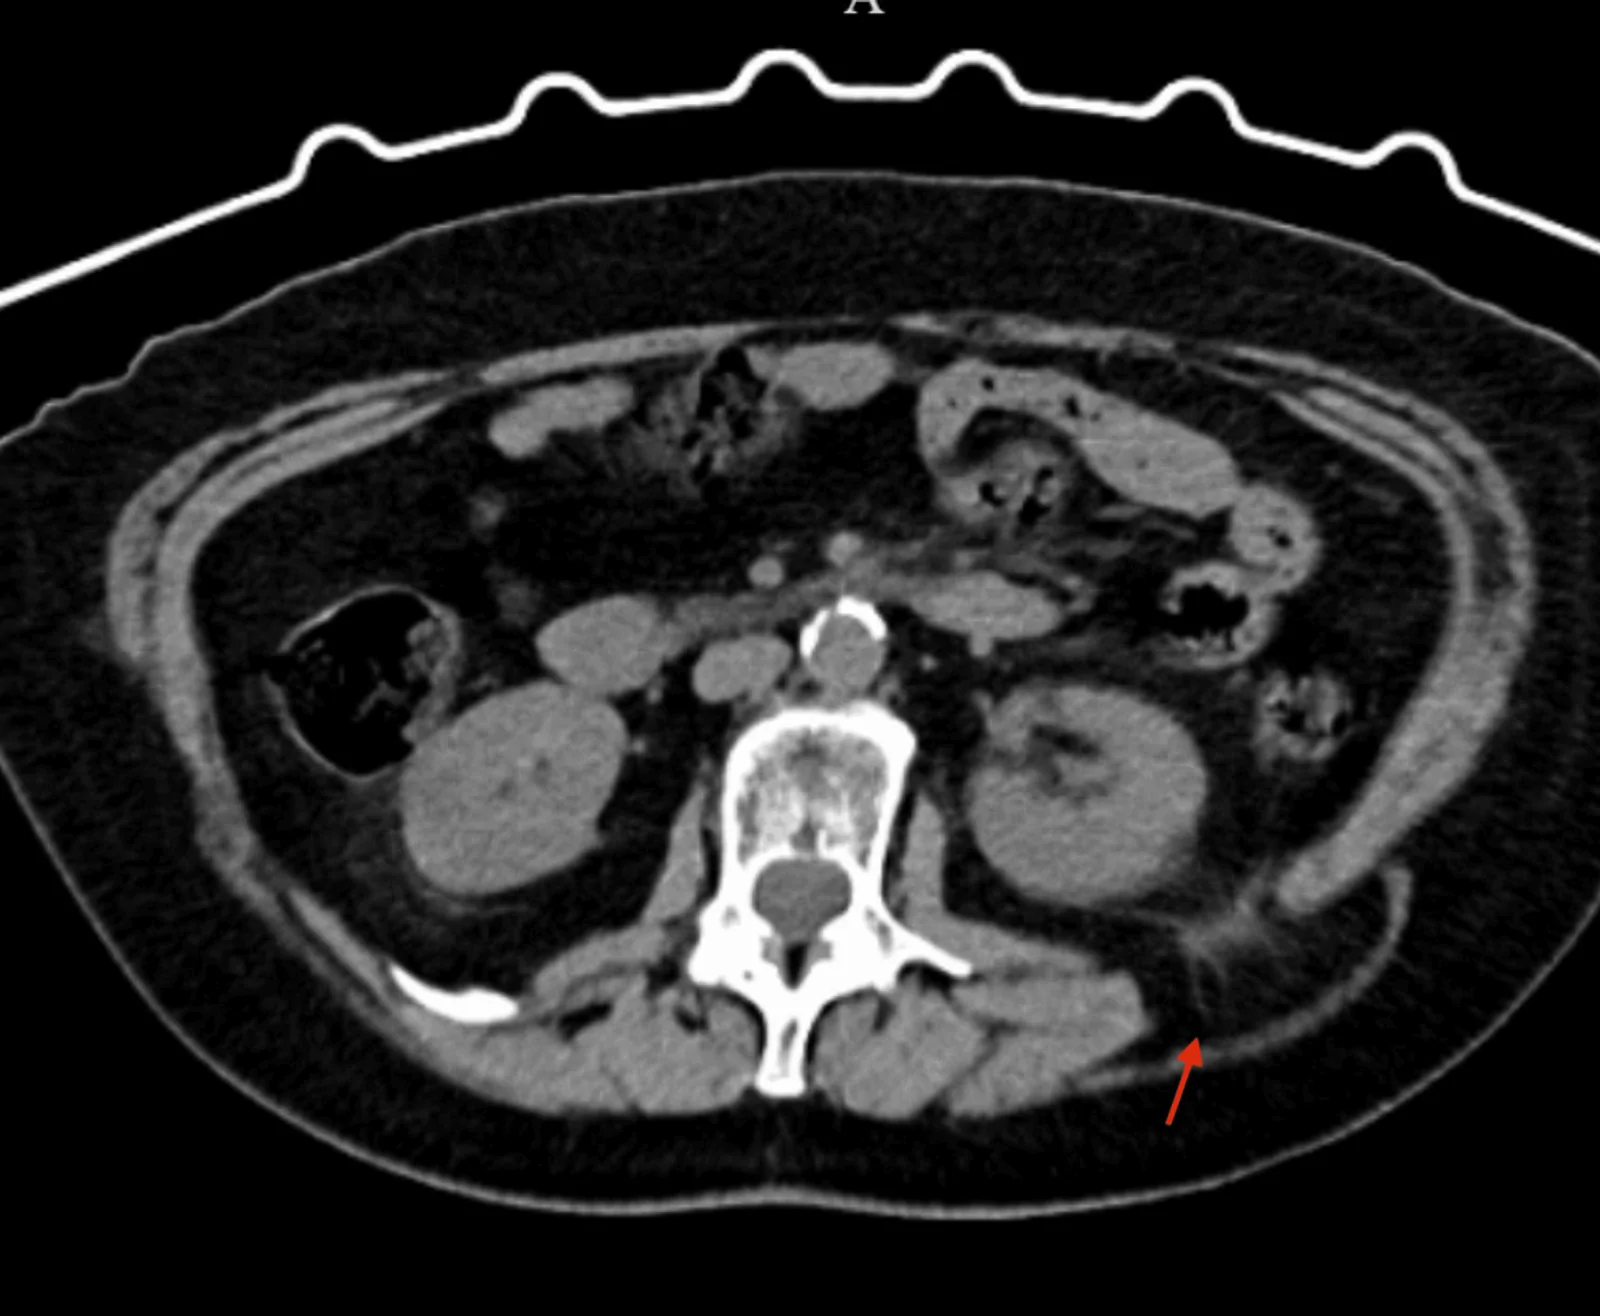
Non-contrast abdominal CT, axial view Superior lumbar hernia defect (arrow) containing retroperitoneal fat without evidence of visceral involvement or incarceration.
